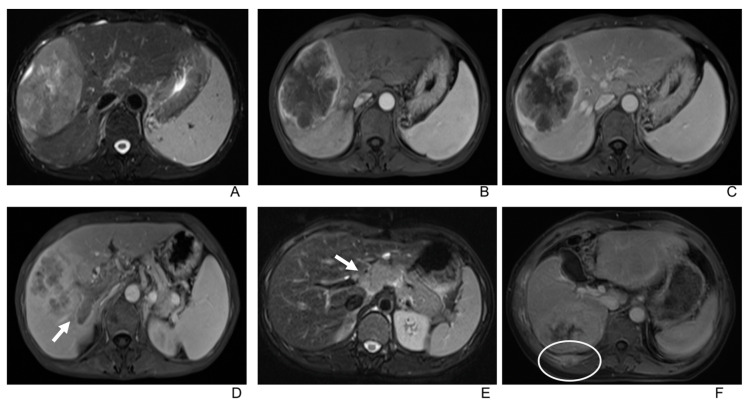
(A) T2-weighted imaging shows uneven hyperintensity of a large intrahepatic lymphoepithelioma-like cholangiocarcinoma in a 55-year-old male. (B,C) Tumor shows peripheral rim enhancement in the arterial phase with centripetal progressive enhancement (Type A enhancement pattern) in the portal venous phase. (D) Tumor thrombosis in the right branch of the portal vein (arrow). (E) Enlarged lymph node in the portal hepatis (arrow) in a 39-year-old female. (F) Metastasis in the 12th rib (circle) of a 56-year-old male.
